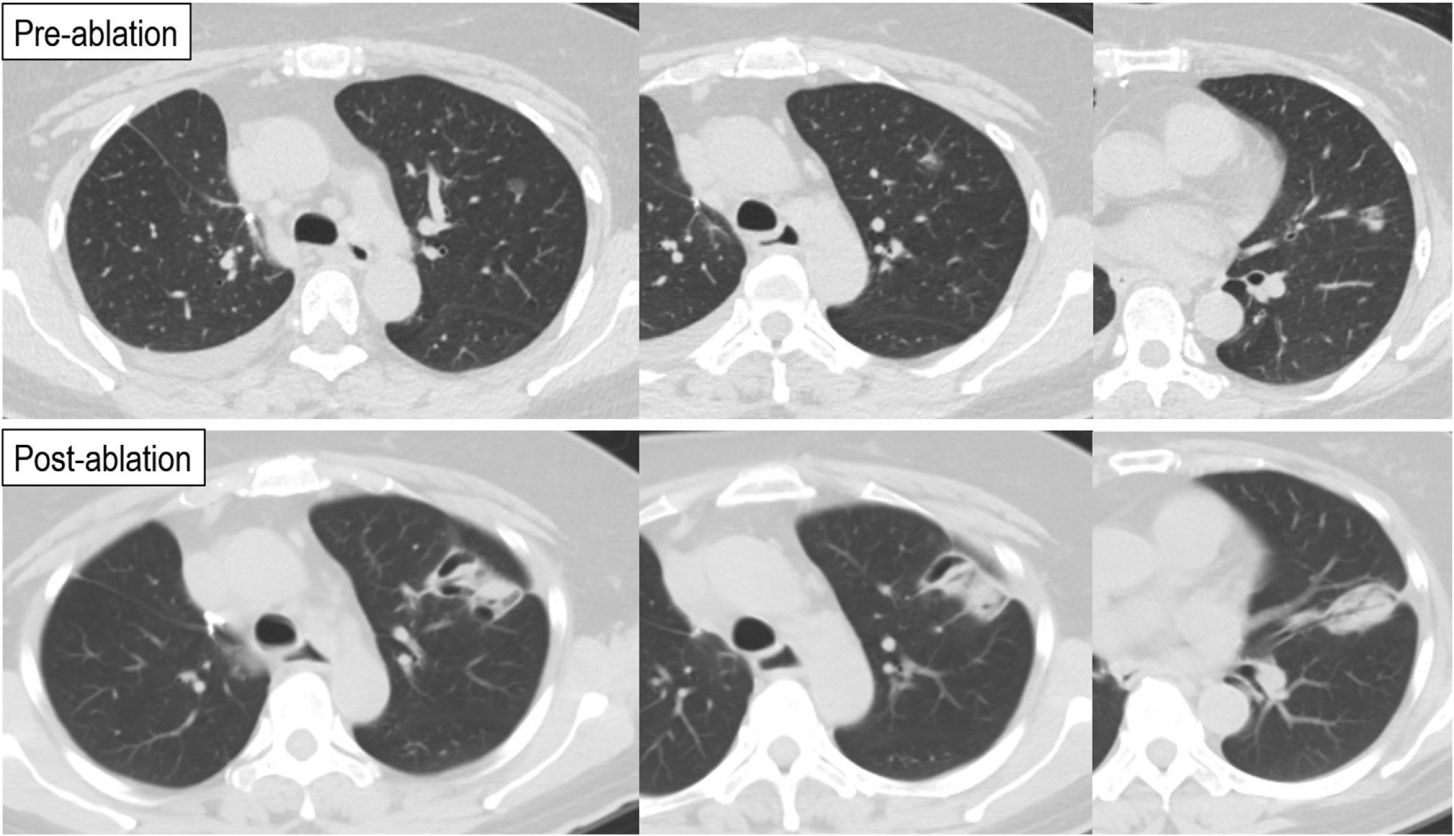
An example of concomitant triple nodule ablation for multifocal lung cancer. The patient had a *right upper* lobectomy and *right lower* lobe wedge for early lung cancer previously. *Top*: preablation CT scans of 3 ground-glass lesions in the *left upper* lobe, which progressed on follow-up. *Bottom*: 1-month postablation CT scans demonstrating 2 closely related and merged ablation zones and another more inferiorly.
